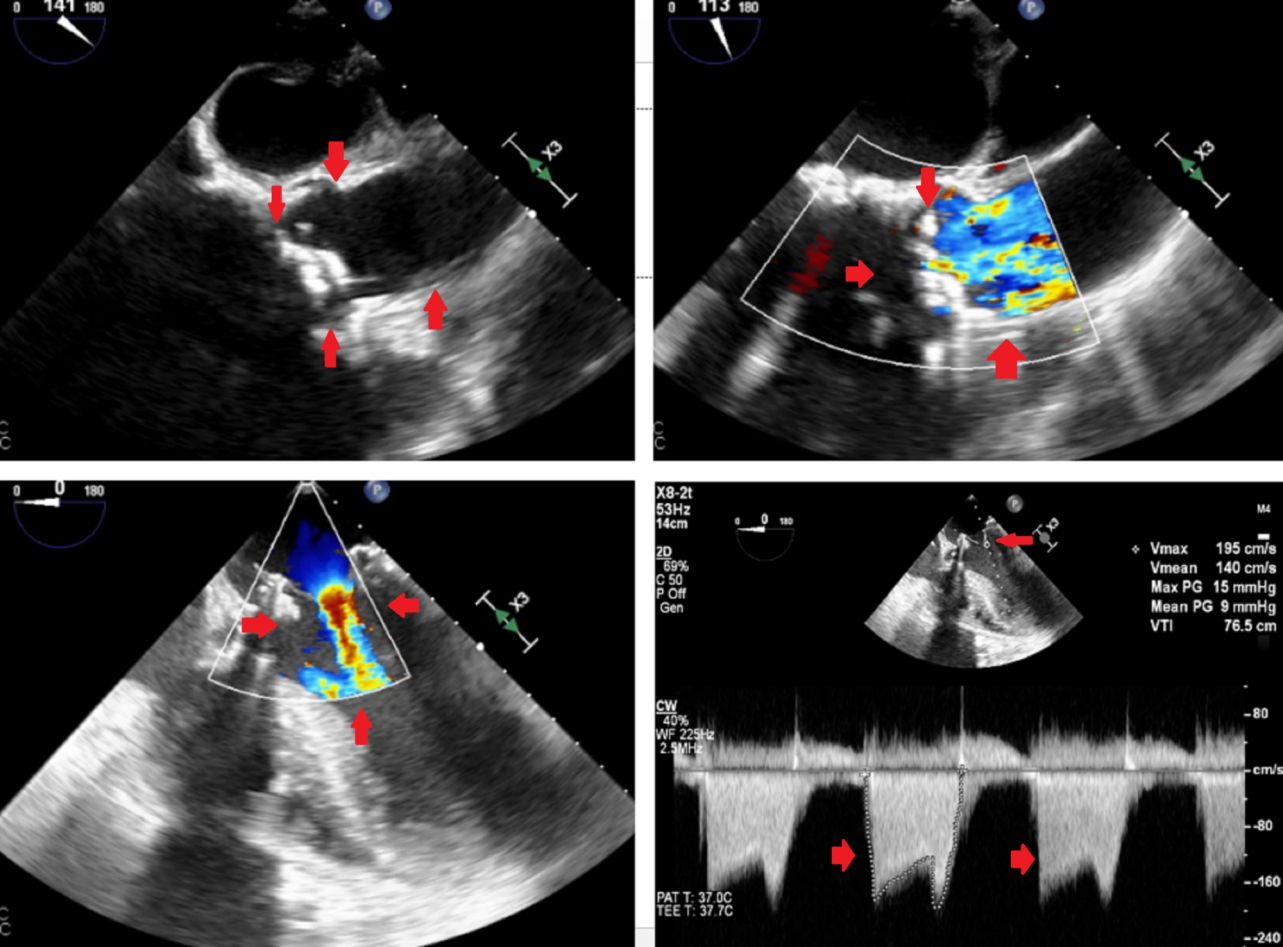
Transesophageal echocardiogram nine days after surgical mitral valve replacement with top left and right showing severe aortic stenosis and elevated gradients across the aortic valve and bottom left and right with elevated gradients across bioprosthetic mitral valve with signs of early dysfunction
